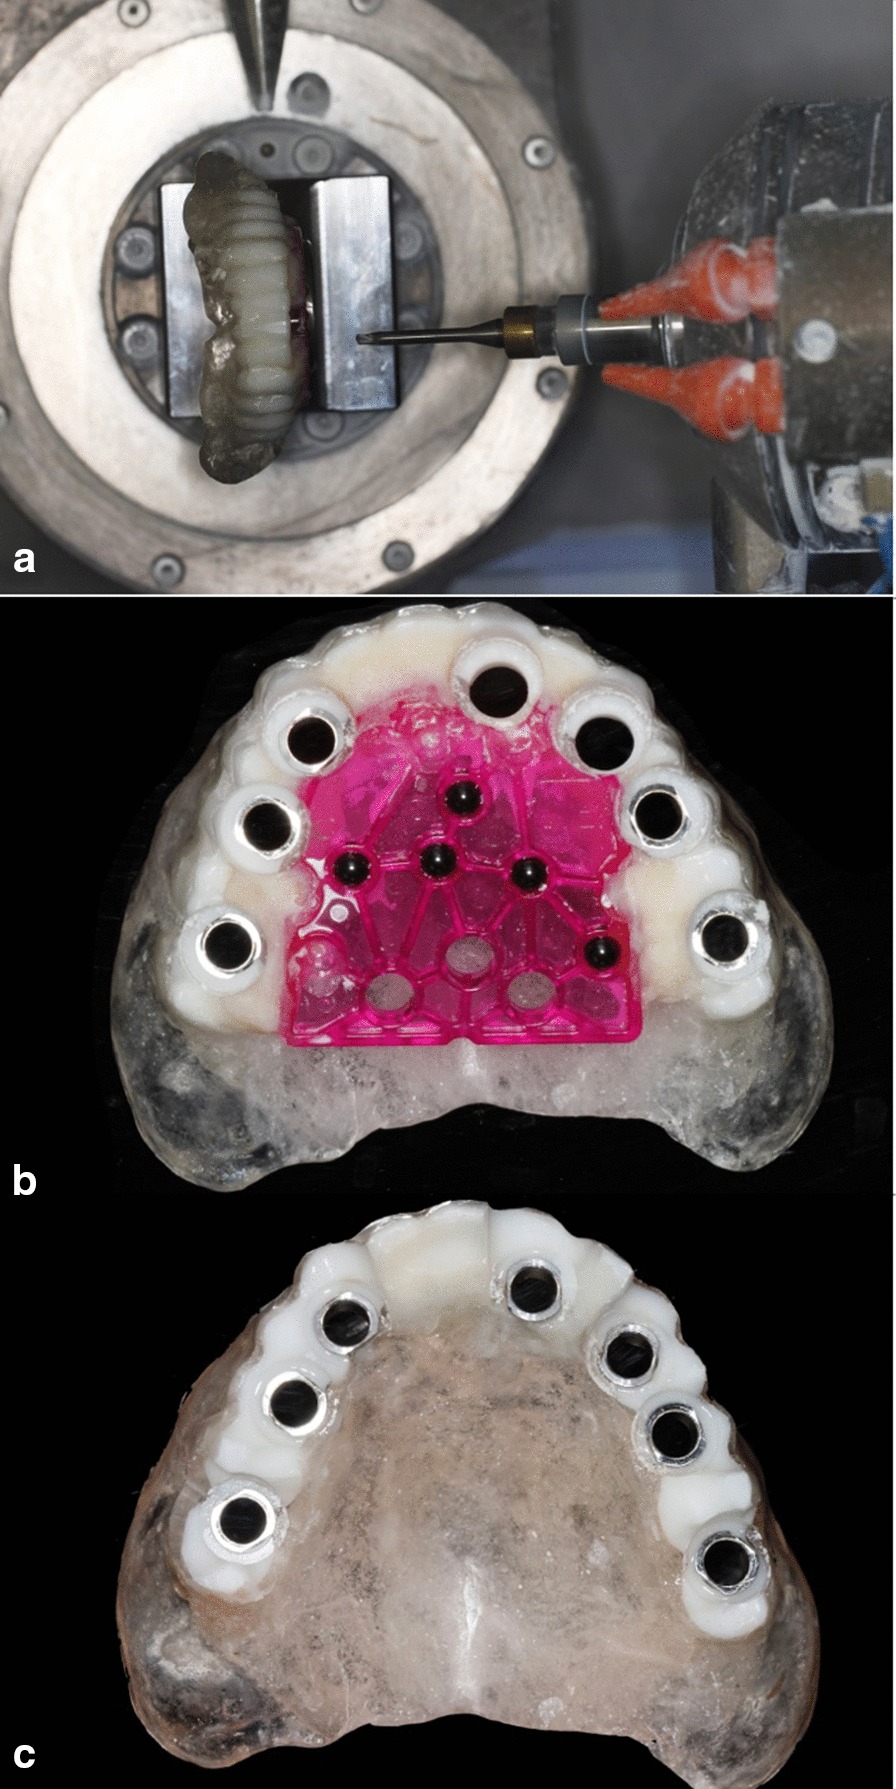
The radiographic template was transferred into the surgical guide by the CNC milling process. a The radiographic template was fixed on the holder of the CNC milling machine, and slots of the sleeves were milled on the radiographic template. b Steel guide sleeves were installed into the slots. c The registration template was removed, and the radiographic template was transferred into a surgical guide
